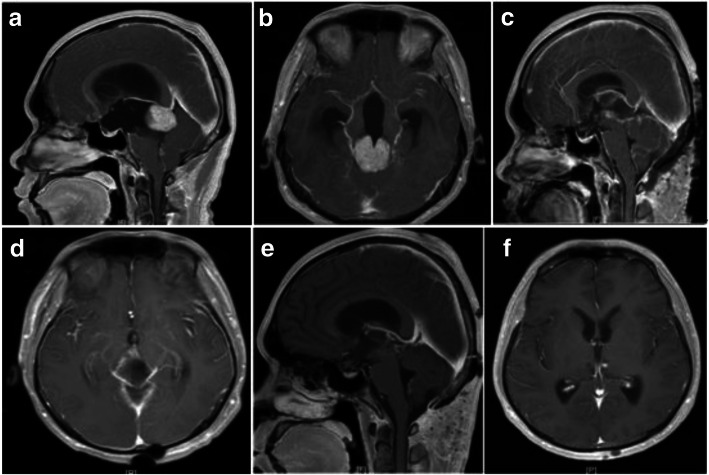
Preoperative and postoperative images of germinoma: a and b preoperative MRI shows tumor in the pineal region; c and d Three days after subtotal resection of the tumor; e and f Three months after operation, the tumor disappeared after radiotherapy and chemotherapy
